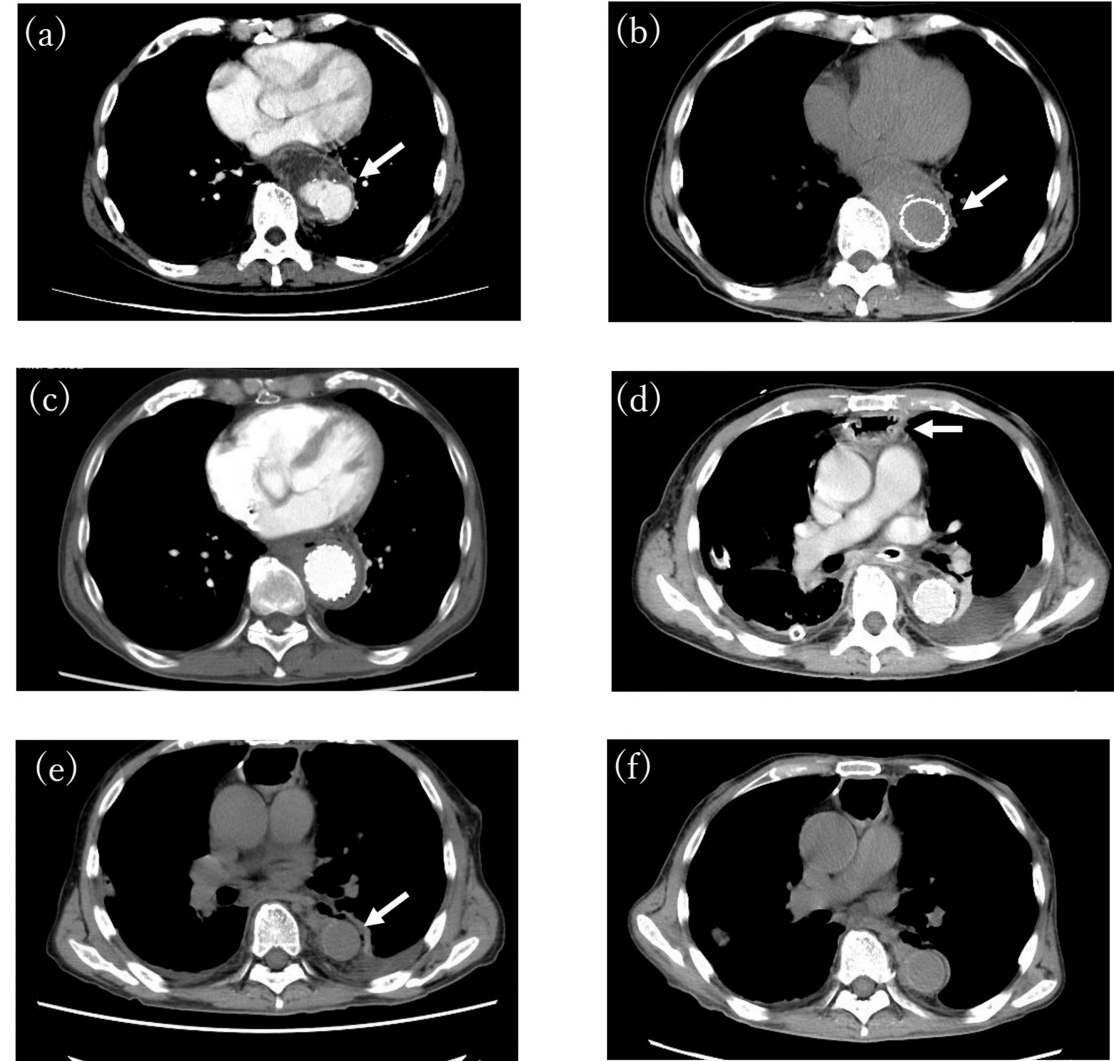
The time course of the axial-plane computed tomography (a) A 60-mm saccular pseudoaneurysm in the descending aorta (arrow); (b) After thoracic endovascular aortic repair (TEVAR) (arrow); (c) Two months after the TEVAR; (d) After esophageal reconstruction (arrow); (e) After descending aorta replacement (arrow)
